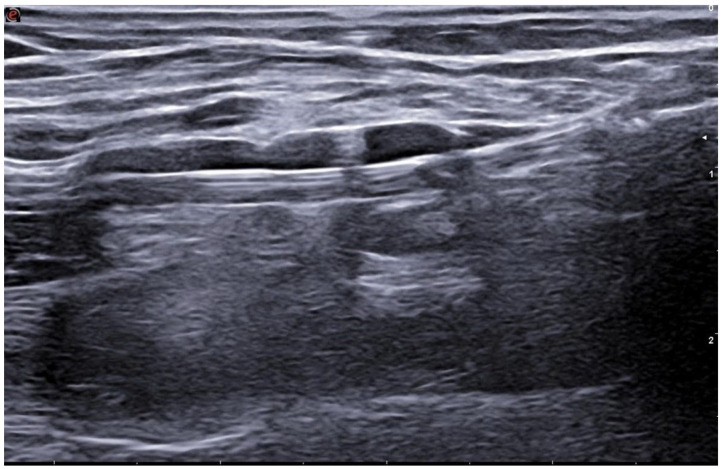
Under ultrasound guidance, the anastomosis is remodeled using 5 mm balloon angioplasty.
